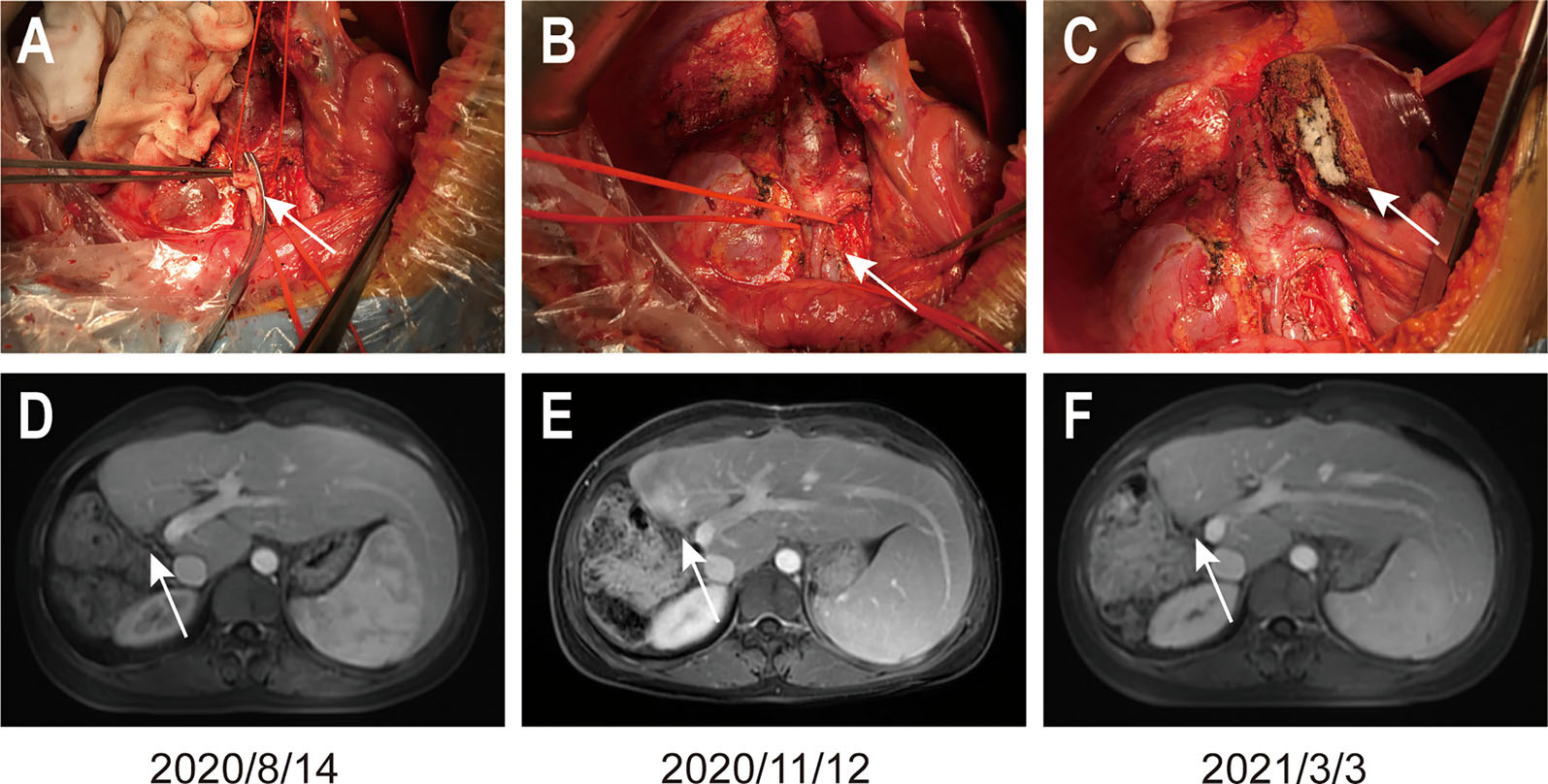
Images of the operation and the MRI reexamination after surgery. (A) shows the inferior vena cava embolectomy, (B) shows the sutured inferior vena cava, and (C) shows the liver incisal margin. Arrows in (A, B) figures indicate the position described above. (D–F) show the MRI reexamination after surgery. Arrows in (D–F) figures indicate the liver resection margin.
